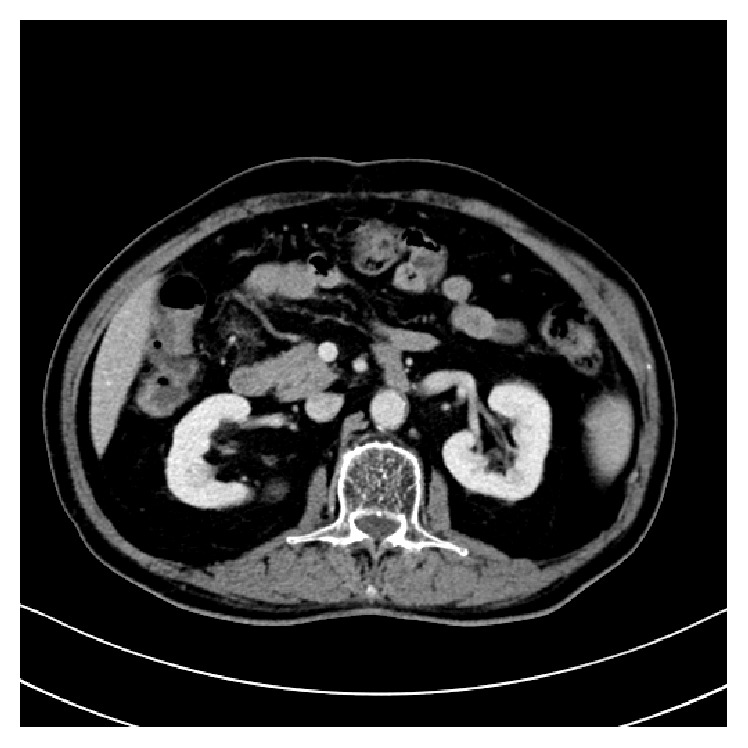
CT imaging performed in 2016. CT scan showed no evidence of para-aortic lymph node recurrence.
